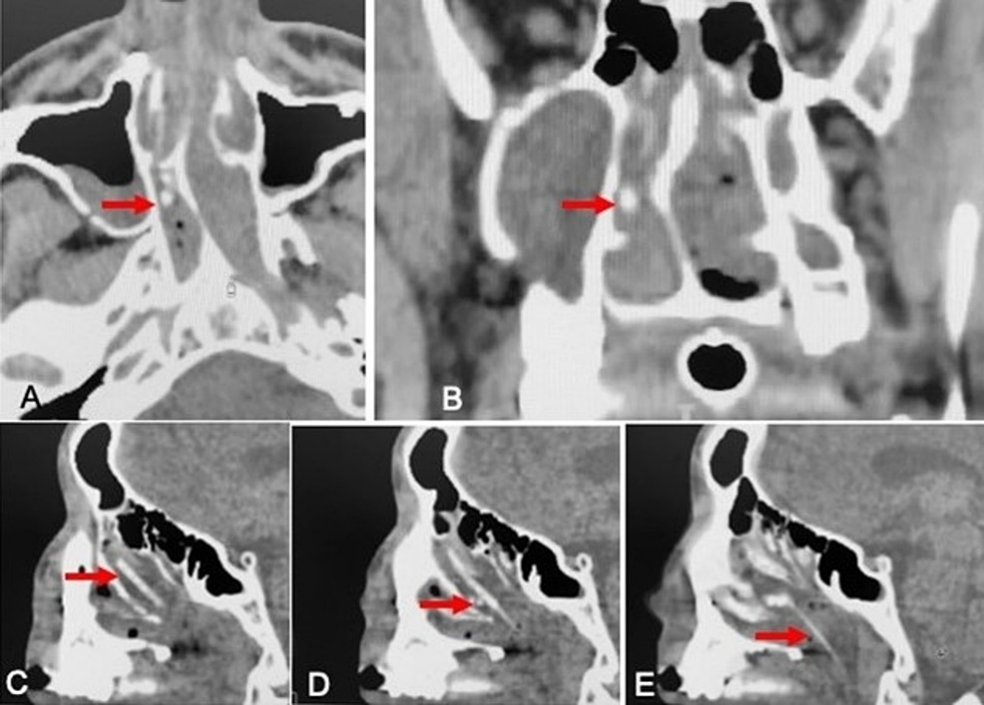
CT scan of the nose CT scan showing the swab (arrow) behind the septal spur in axial and coronal view (A,B) and the entire swab in the sagittal projection (C,D,E) reaching the nasopharynx and after rupture dislocated in the upper part of the nose and also migrated below the middle turbinate.
